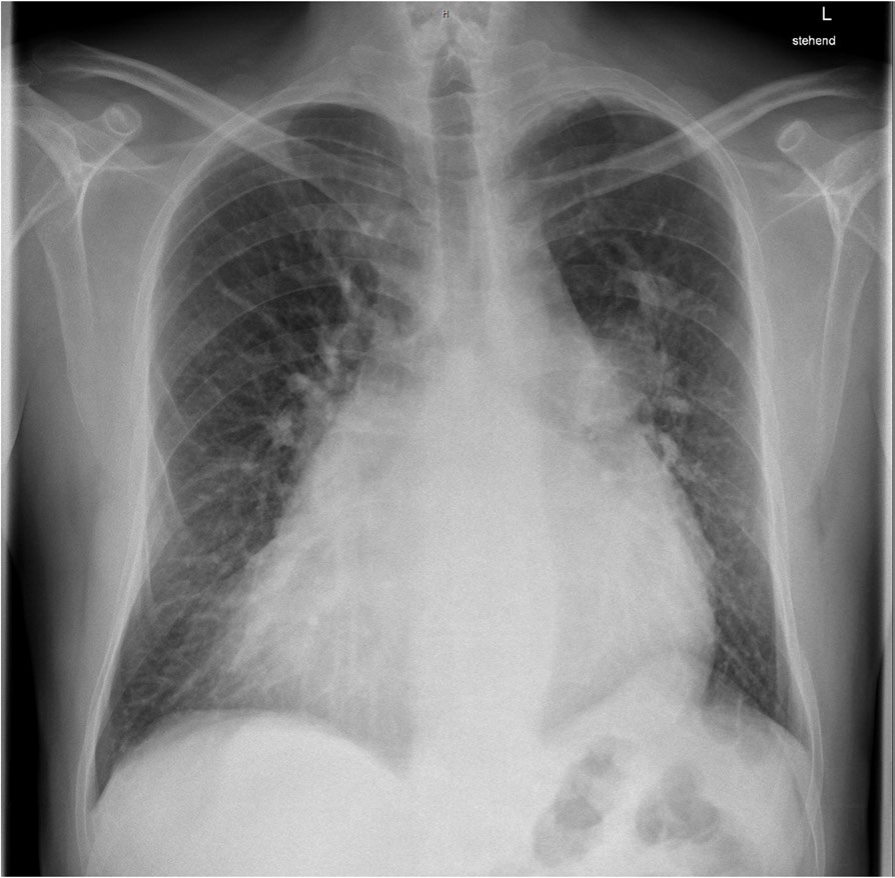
Preoperative chest X-ray of a 46-year-old man with congenital heart disease shows dextrocardia, cardiomegaly and no opacities or pleural effusion
